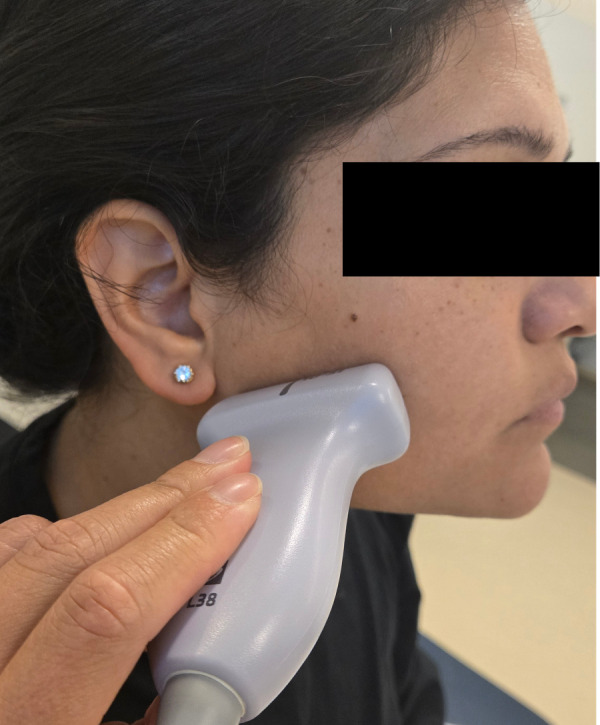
Transverse scanning plane of the parotid gland using point of care ultrasound (POCUS).
